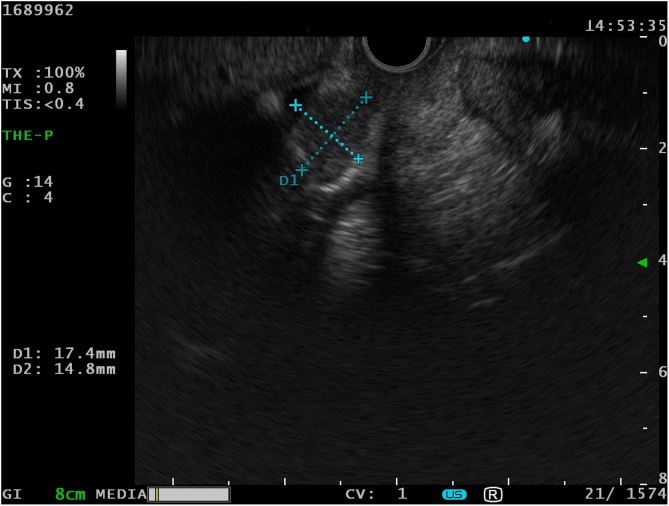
Endoscopic ultrasound image showing the hypoechoic mass of 17.4 mm × 14.8 mm.
